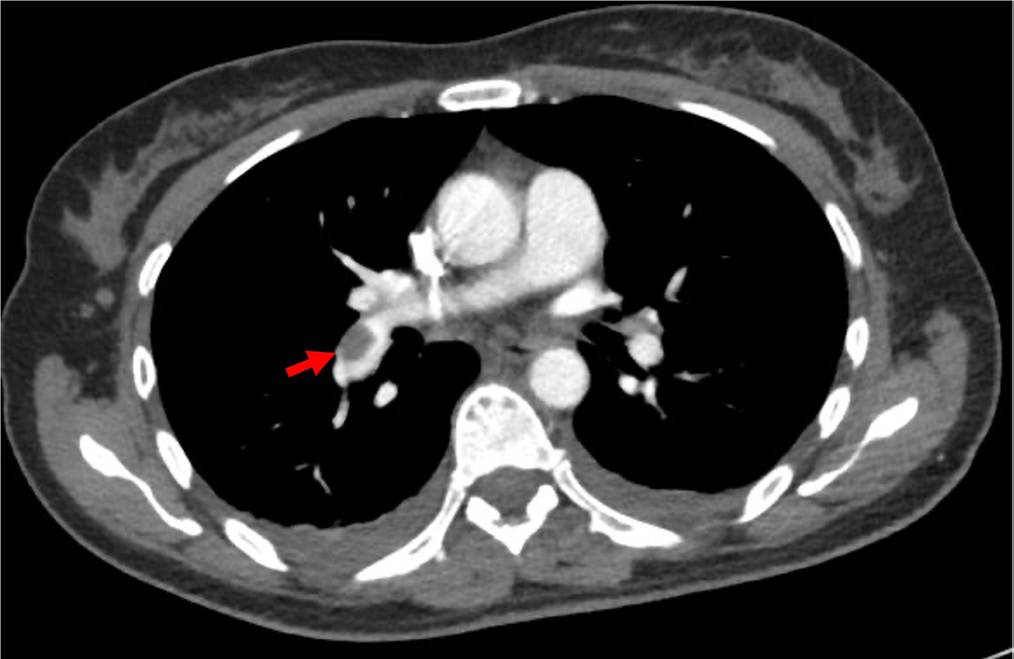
CT pulmonary angiogram demonstrating embolus in the right lower lobe pulmonary artery (arrow).
